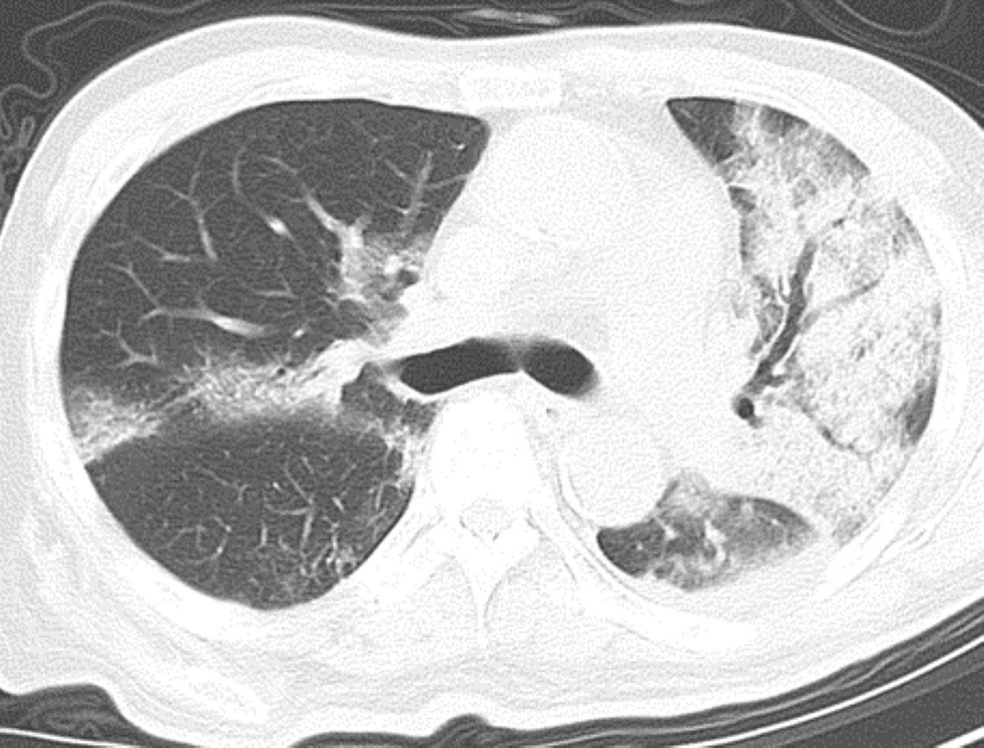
The unenhanced computed tomography (CT) scan that was taken three days before admission at another hospital The CT scan showed multiple patchy ground glass opacity in the subpleural area of both lungs and consolidation and air bronchogram sign on the left upper lobe of the lung
